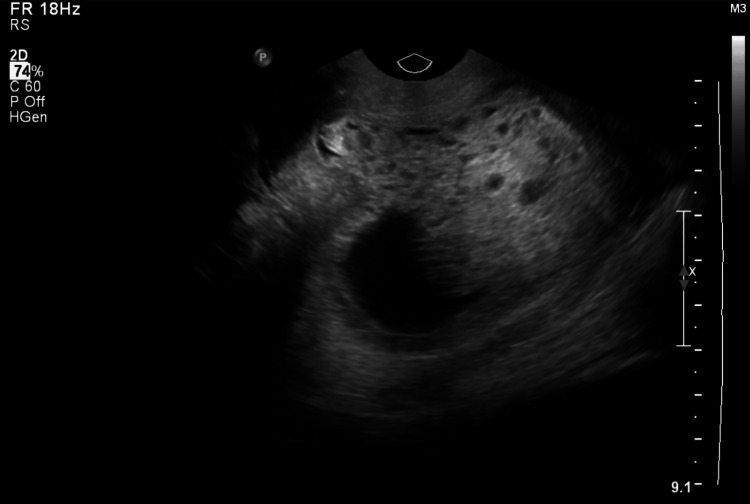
Ultrasound at nine weeks showed no fetal pole with complex echogenic intrauterine masses containing multiple cystic areas
